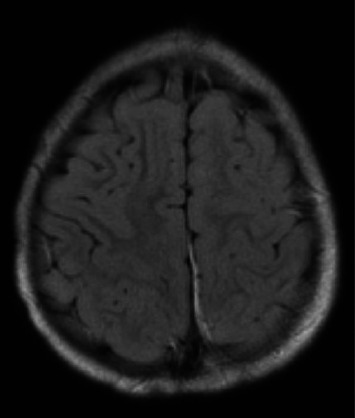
MRI head without IV contrast. Abnormal signal in the left occipital lobe may represent a venous anomaly and chronic blood products but other etiologies not excluded.
